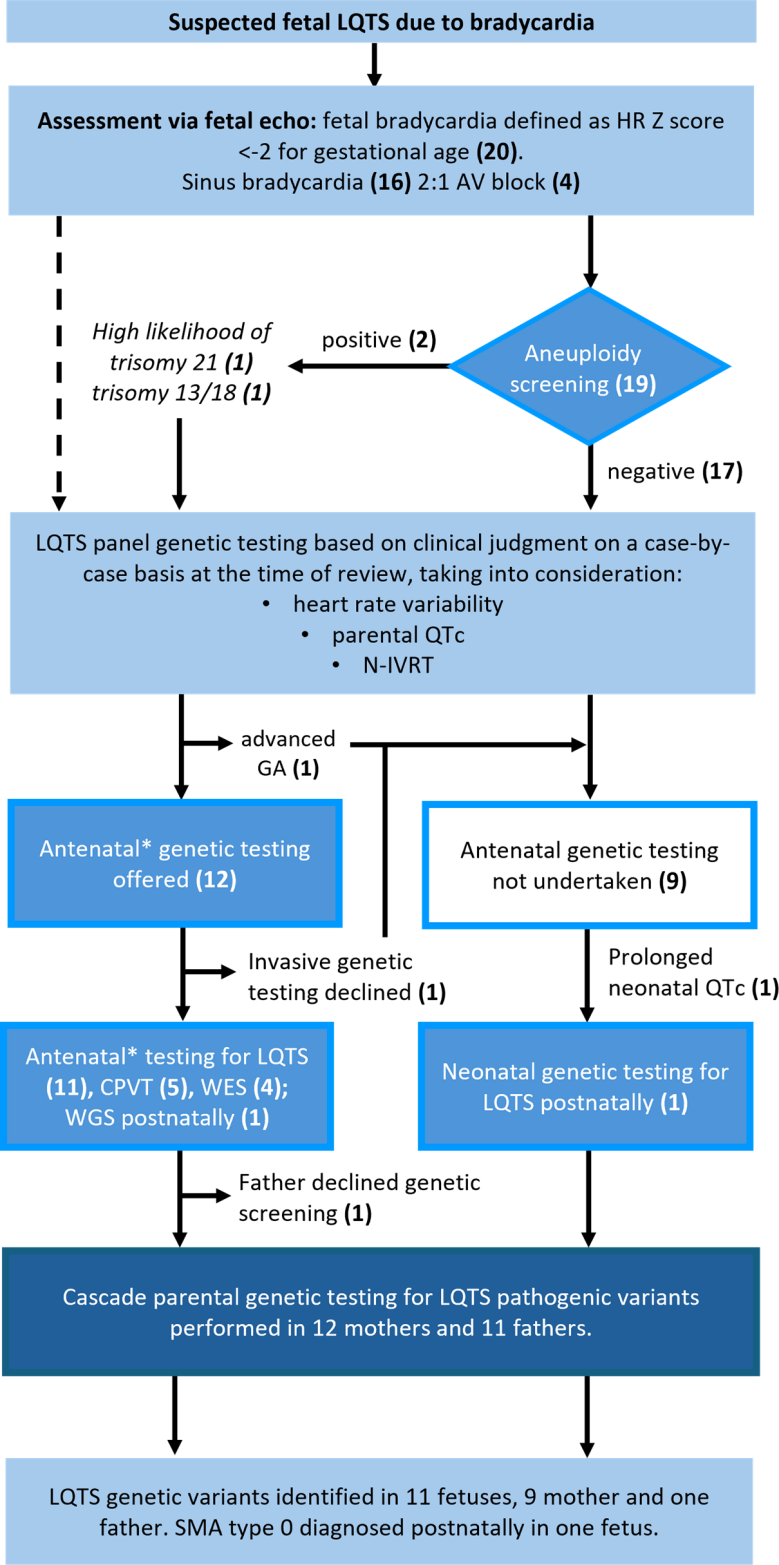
Summary of our assessment of fetuses with persistent bradycardia. AV indicates atrioventricular; CPVT, catecholaminergic polymorphic ventricular tachycardia; echo, echocardiogram; GA, gestational age; HR, heart rate; LQTS, long QT syndrome; N-IVRT, isovolumetric relaxation time as an index of the cardiac cycle; QTc, corrected QT interval; SMA, spinal muscular atrophy; WES, whole exome sequencing; and WGS, whole genome sequencing. *Antenatal genetic screening involved either amniocentesis or testing maternal blood.
